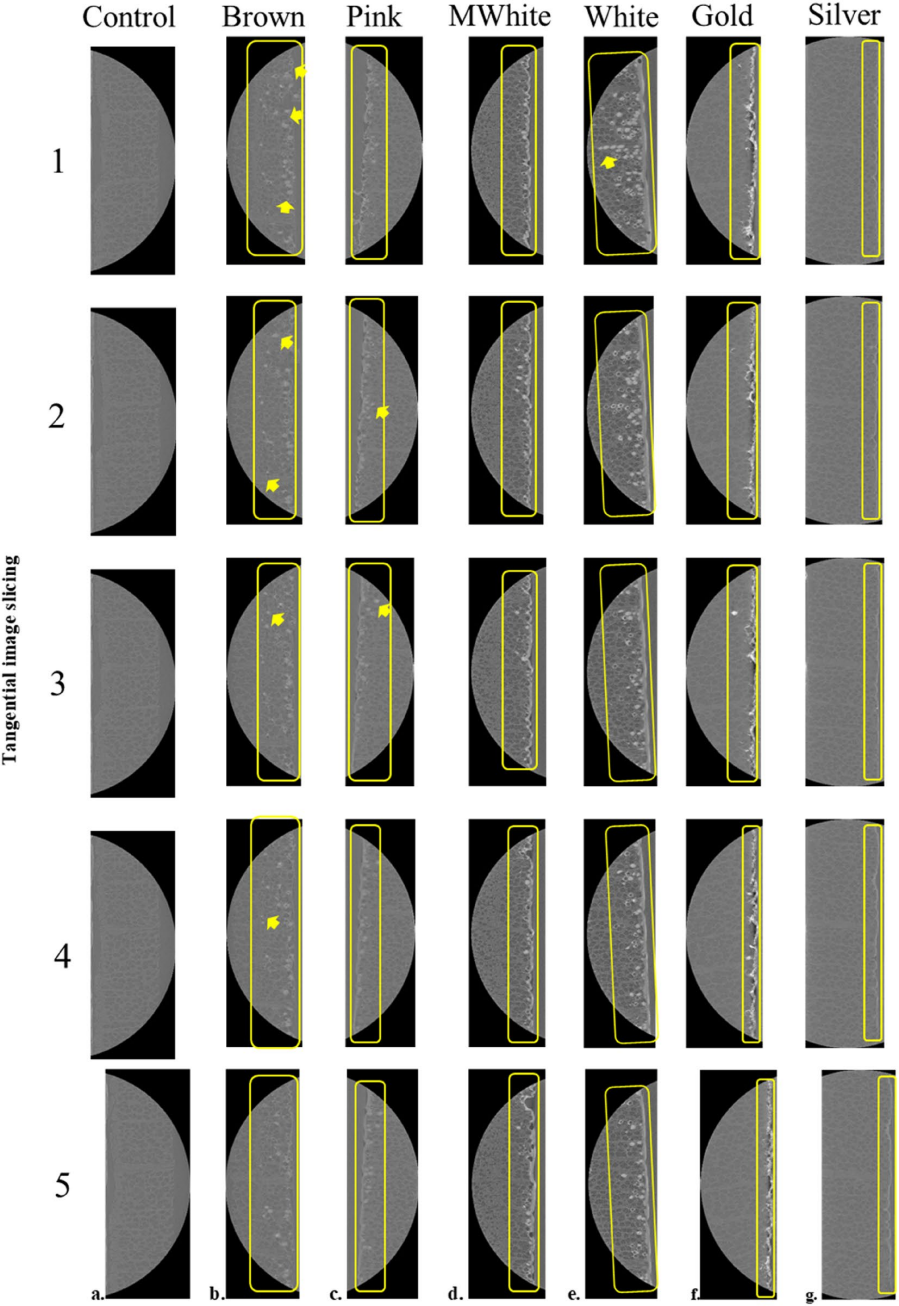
The 2D X-ray visualization of various coatings in transverse section from tangential image slicing. (a) Control (no treatment), (b) brown coating, (c) pink coating, (d) matte white coating, (e) white coating, (f) gold coating, (g) silver coating; yellow arrow indicating the coating existence in the wood vessels, yellow line indicating the coating area on the surface.
